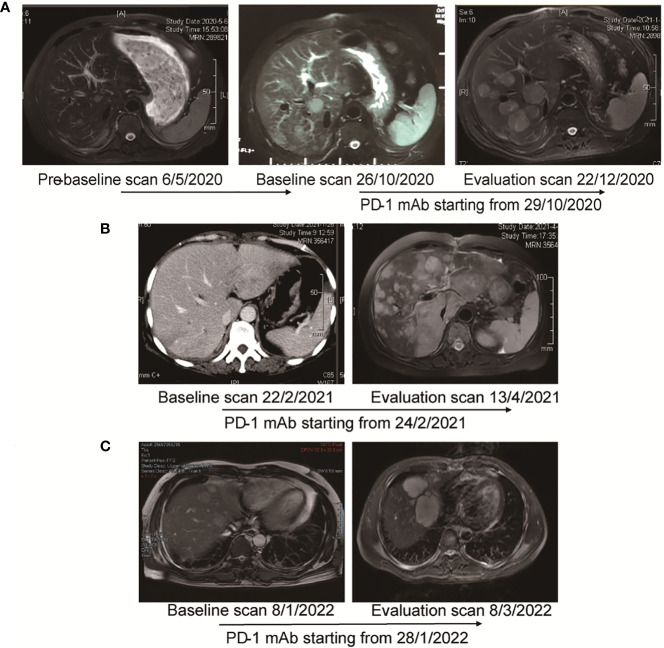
MR images and the timeline of therapy in three cases with HPD. (A) Case 1. Pre-baseline MR was obtained before ablation. After confirmed progression 5 months later, the patient adopted immunotherapy. Evaluation scan after 1.8 months showed significant radiological progression of disease with occurrence of new lesions. The TGR fold was calculated to be 11.90. (B) Case 2. After 2 cycles of immunotherapy, MR showed significant progression of intra-hepatic lesions. TTF was 1.6 months. TBI was 579.5%. (C) Case 3. After 2 cycles of treatment, MR scan showed significant progression of disease with increase in size of hepatic lesions. TTF was 1.3 months. TBI was 53.5%.
